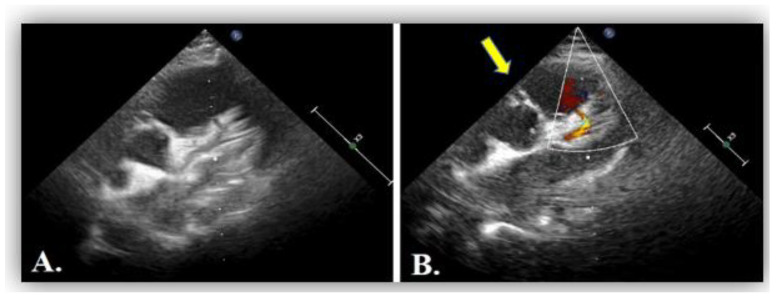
(A) Transthoracic echocardiography in parasternal short axis view showing dilated left coronary artery, LCA. (B) Artefactuous image of the right coronary artery showing the apparently normal origin of the right coronary artery from the aorta at the 11 o’clock position (yellow arrow).
